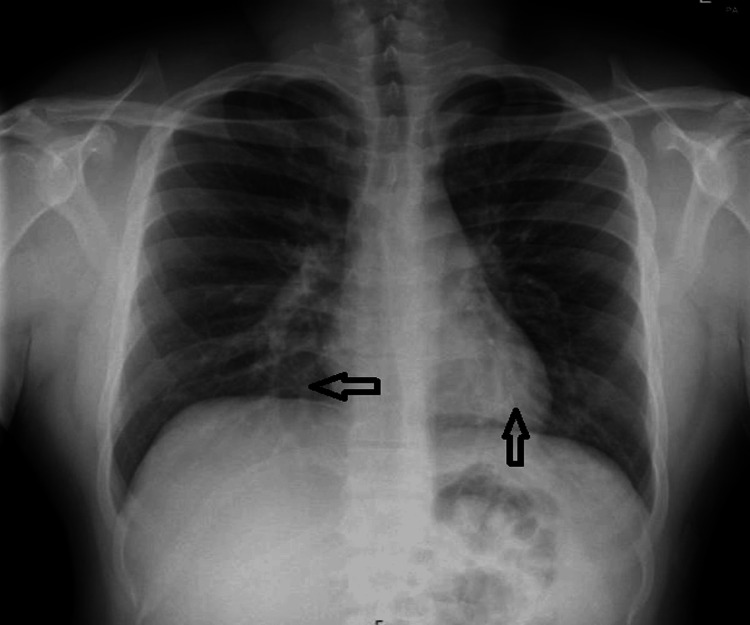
Chest X-ray: bilateral nonspecific inflammatory changes
